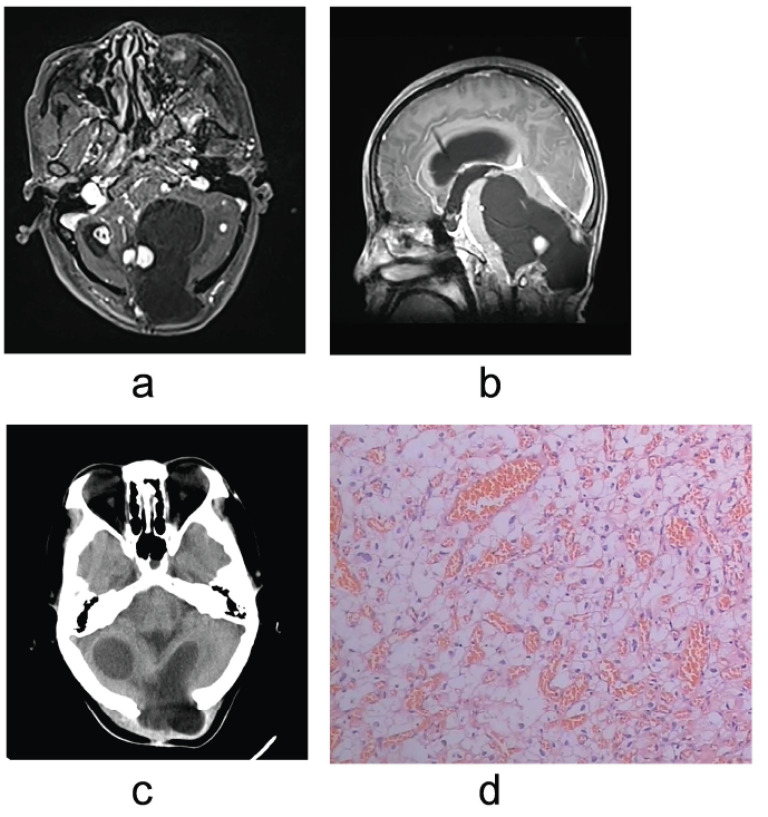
(a) and (b): A preoperative enhanced MRI showed multiple cystic and solid lesions within both cerebellar hemispheres, resulting in compression of the fourth ventricle. (c): A postoperative computed tomography scan performed 5 days after surgery showed a decrease in the size of the cystic lesion, especially in the left cerebellar. (d): Hemangioblastoma was verified *via* pathological examination.
